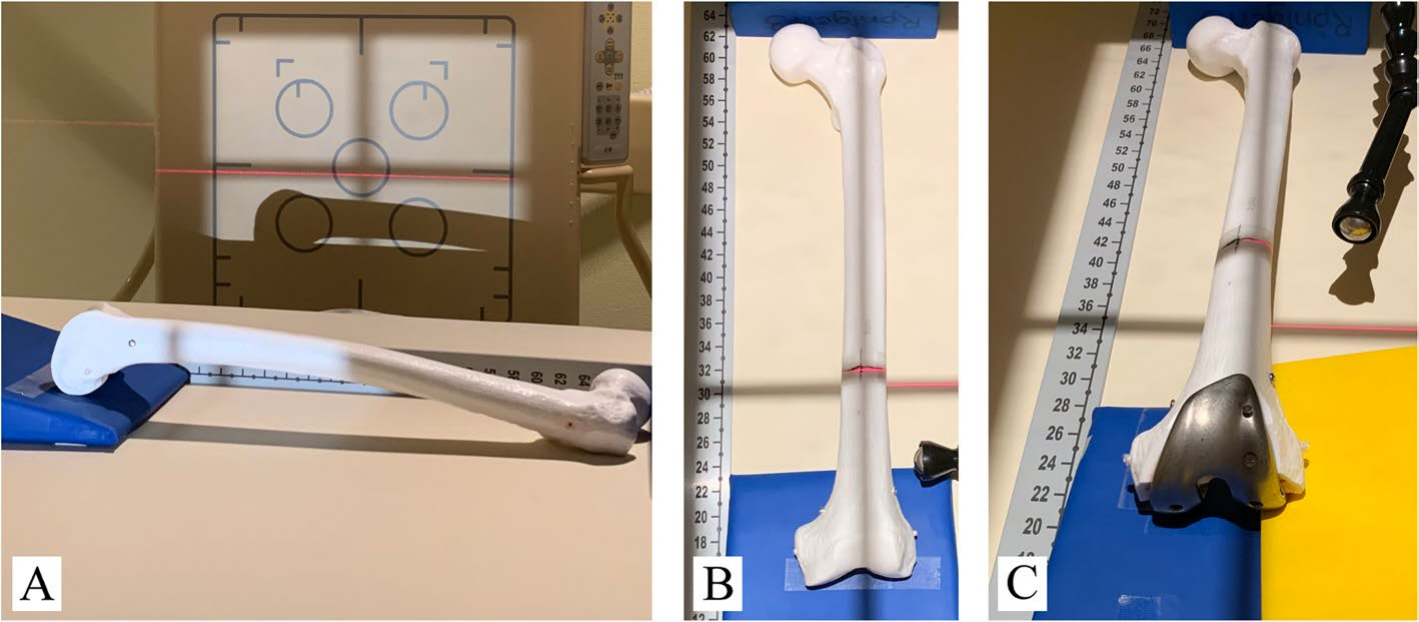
Experimental setup of radiographic imaging with validation and setup of differenct error conditions. Flexion error validation in true lateral view (a). A.p. view preoperative with 5° flexion error (b). A.p. view postoperative with internal rotation error (c). Laser is used to verify bone position on X-ray table. Calibration bar and calibration ball for internal validation of distance measurement in PACS software
